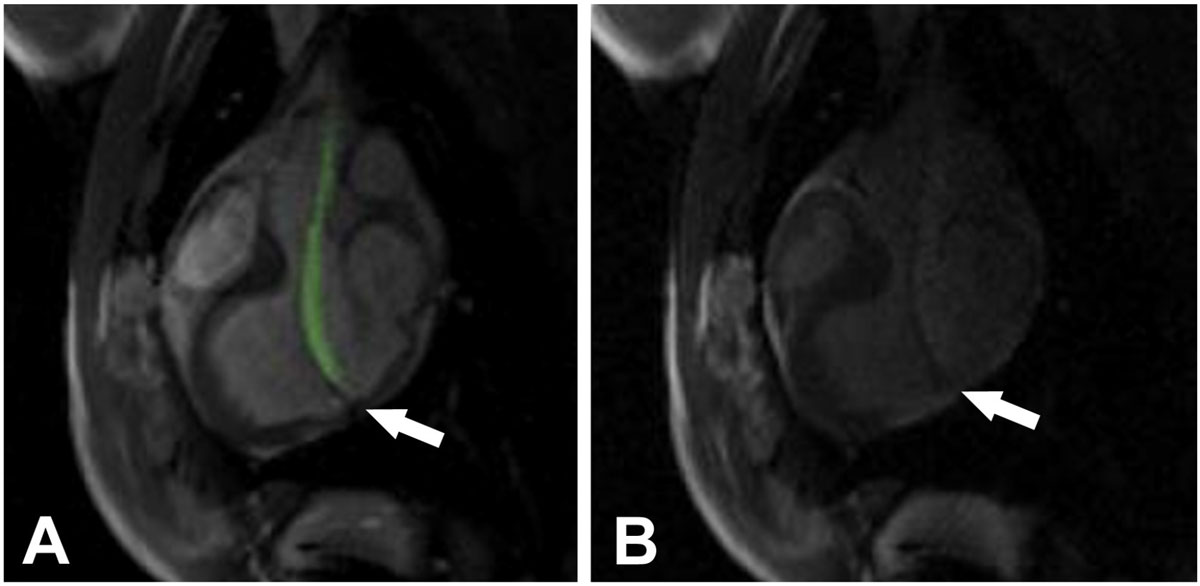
Real-time MRI guided endomyocardial biopsy using an active visualization bioptome.
